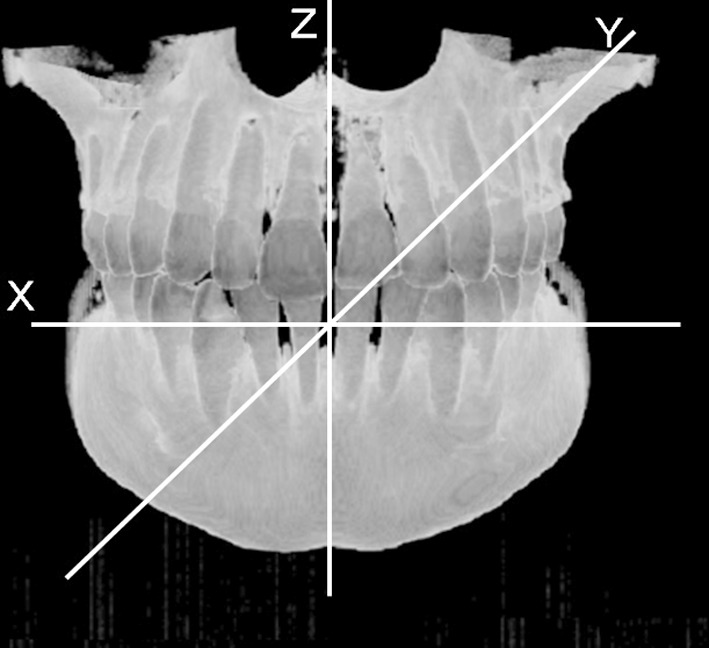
Cone-beam computed tomography image and the three-dimensional coordinate system
